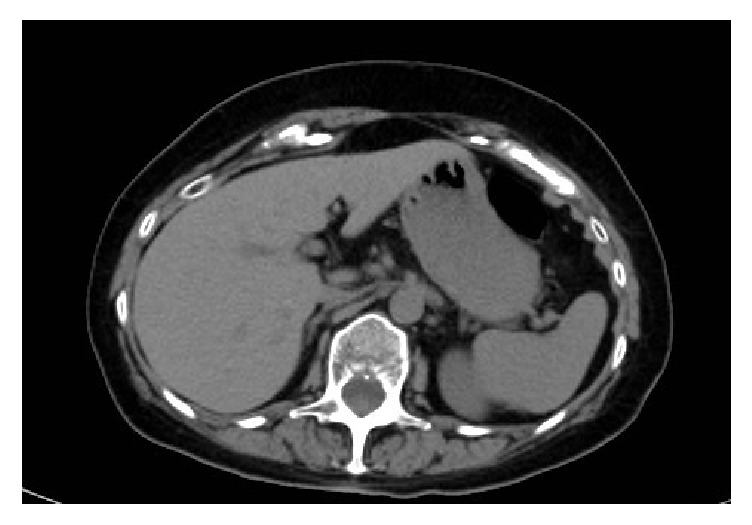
Abdomen CT showing adrenal glands normalized in volume 6 months later.
